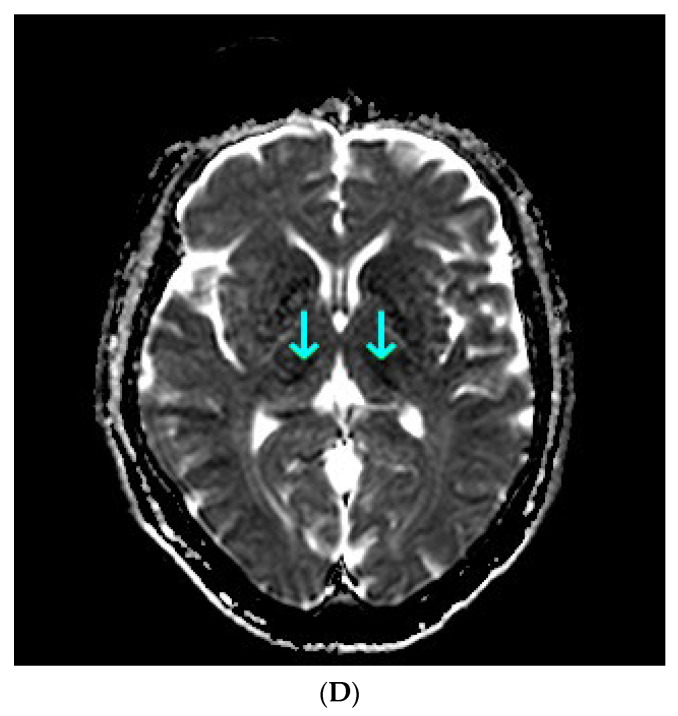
(A) Magnetic resonance imaging of the head. T1 Spin Echo, axial. Green arrows point to hyper-intense globi pallidi; (B) Magnetic resonance imaging of the head. T2 Turbo Spin Echo Dark-fluid. Red single arrows point to the mild symmetric hyper-intensity in the insulas; double red arrow point to the pronounced hyper-intensity in the left occipital lobe; (C) Magnetic resonance imaging of the head. Diffusion Weighted Imaging b = 1000, axial. Cyan arrows point to the restricted water diffusion in both thalami; (D) Magnetic resonance imaging of the head. Apparent Diffusion Coefficient, b = 0, b = 1000, axial. Cyan arrows point to the restricted water diffusion in both thalami.
